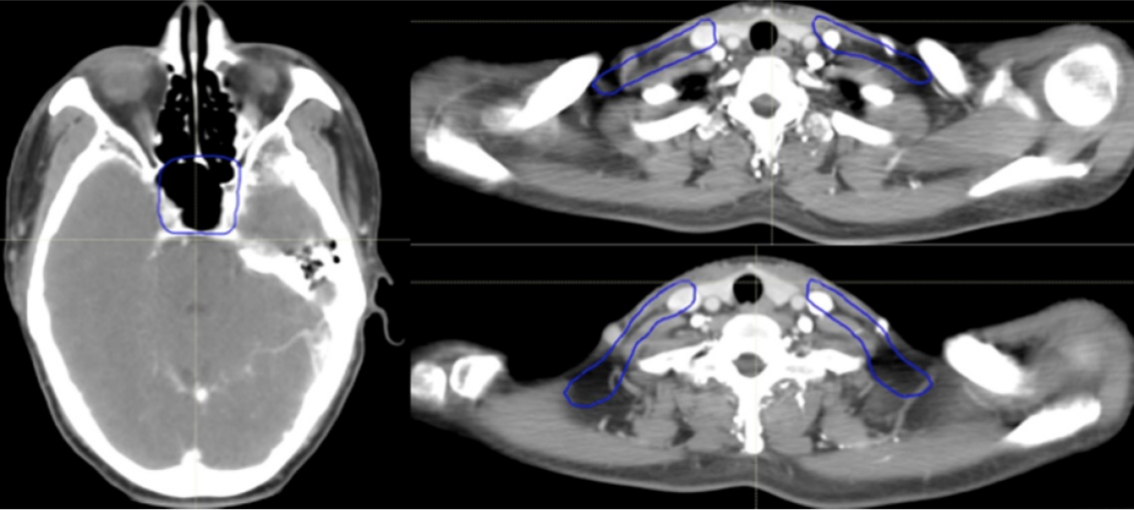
Selected computed tomography slices to demonstrate the delineation of low-risk target volumes. Red: PGTVnx; yellow: PGTVnd; green: PTVnx; pink: PTVna; blue: PTV1. Abbreviations: PGTVnx: planning target volume of nasopharyngeal GTV; PGTVnd: planning target volume of GTV in cervical lymph nodes; PTVnx: planning target volume of nasopharynx; PTVna: planning target volume of neck area; PTV: planning target volume 1.
